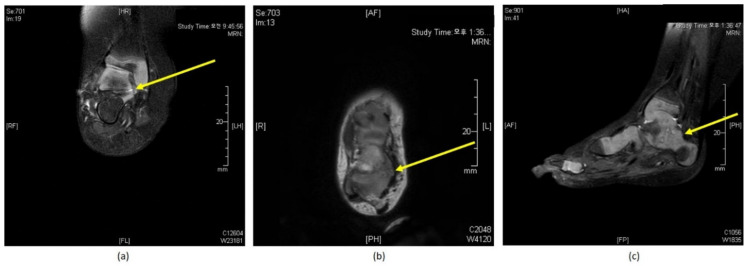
Non-contrast magnetic resonance imaging (MRI) showing talocalcaneal coalition in (a) coronal, (b) axial, and (c) sagittal planes.
